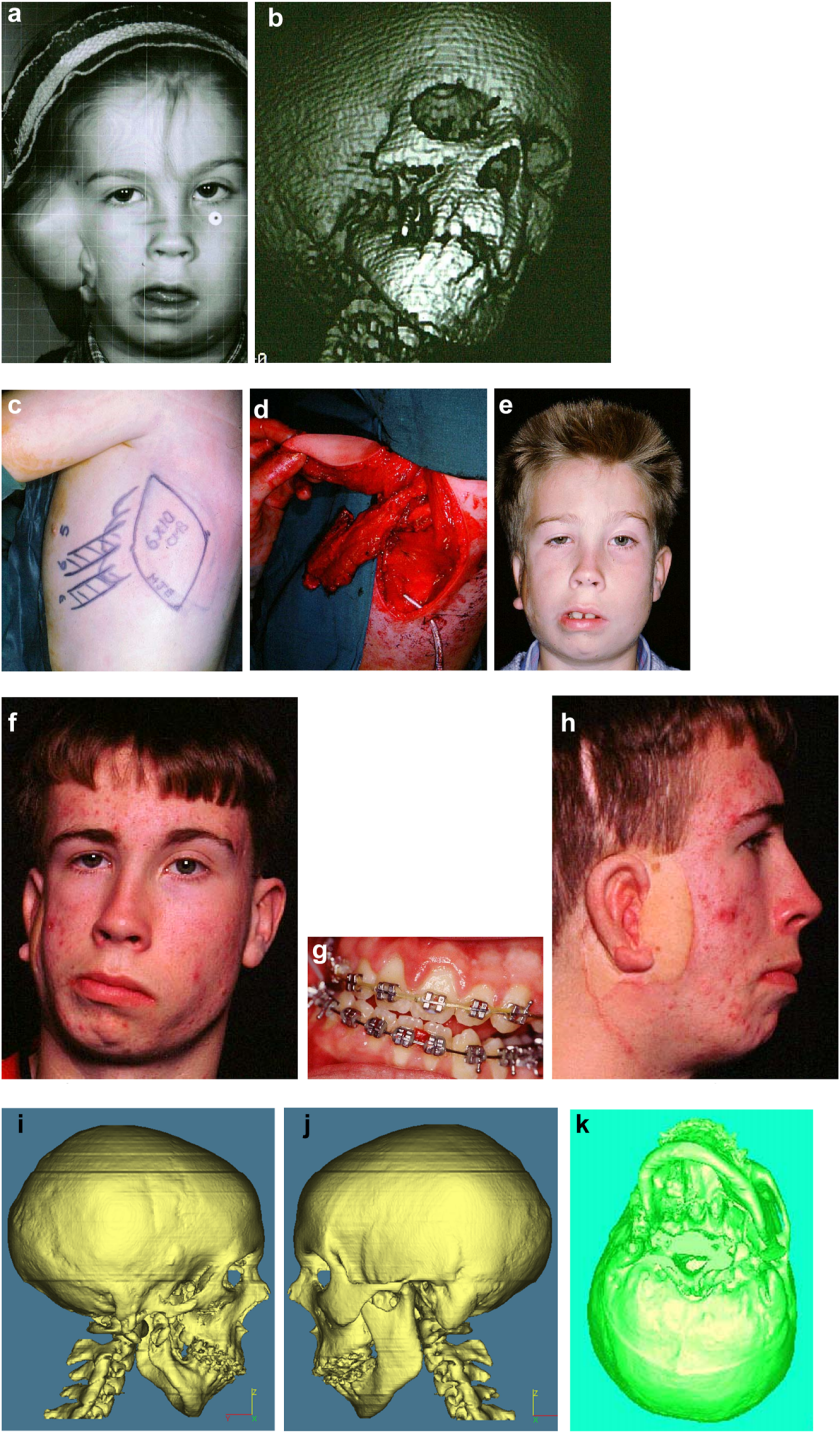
Planning for end stage reconstructrion with bimaxillary rotational osteotomies. (a and b) 8 yr old young man with PK III HFM and no zygomatic arch, glenoid fossa and mandibular condyle. Tissue expander *in situ* to produce sufficient soft tissue for ear construction. 3DCT scan at that age. (c–e) At 9 yrs of age, zygomatic arch, glenoid fossa and mand. condyle constructed with two vascularised ribs and serratus anterior muscle. (f–h) Same patient 9 years later showing established asymmetry. (i–k) Same patient’s 3D CT scan 9 years later. Significant asymmetry has developed again and pre-surgical orthodontics is completed.
